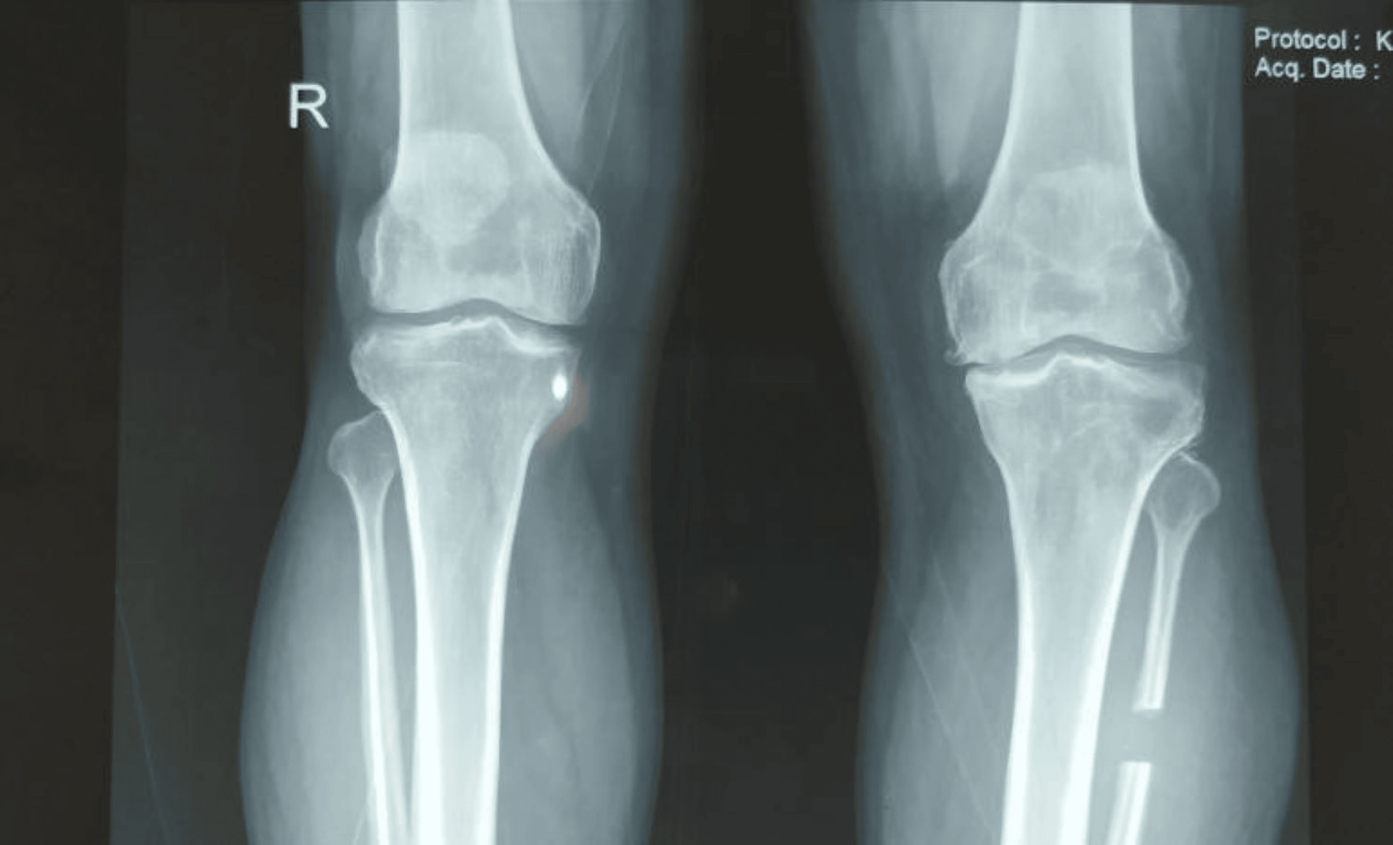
Postoperative AP radiograph showing resection of proximal fibular segment AP: anteroposterior
